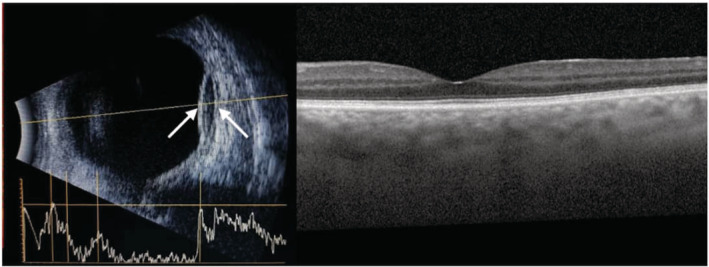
Left, the B‐scan ocular ultrasound image of Case 1 obtained 2 days after the surgery indicating the area of the suprachoroidal hemorrhage (between arrows). Right, EDI‐OCT image of the same patient took 3 weeks after the operation, showing normal macula with a subfoveal choroidal thickening.
